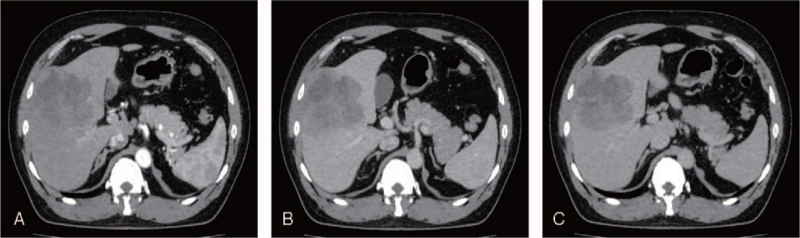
Three-phase enhanced CT of the abdomen. (A) Arterial phase CT image showed irregular patch-like enhancement; CT images in (B) the portal venous phase, and (C) the delayed phase.
